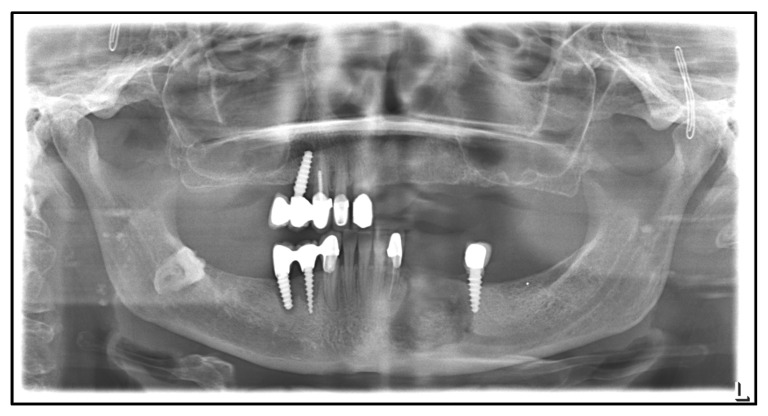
Dental X-ray at the time of admission with extended MRONJ of the left anterior mandible and ongoing intraoral fistulation, January 2020.
